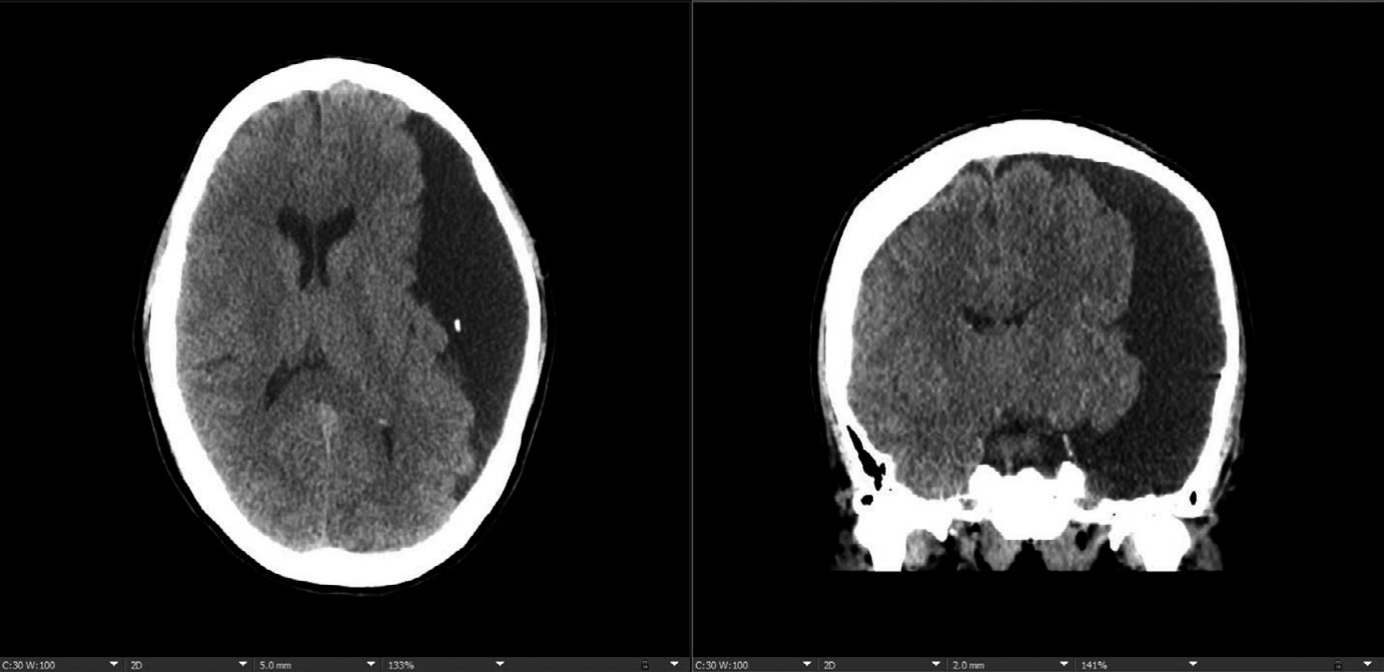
Computed tomography images of the head in axial (left) and coronal views (right)—showing large CSF density extra‐axial fluid collection in the left hemisphere measuring 12 cm × 11 cm × 4.5 cm in size along the left cerebral convexity. There is no communication with the ventricular system with smooth scalloping of the calvarium on the left side
